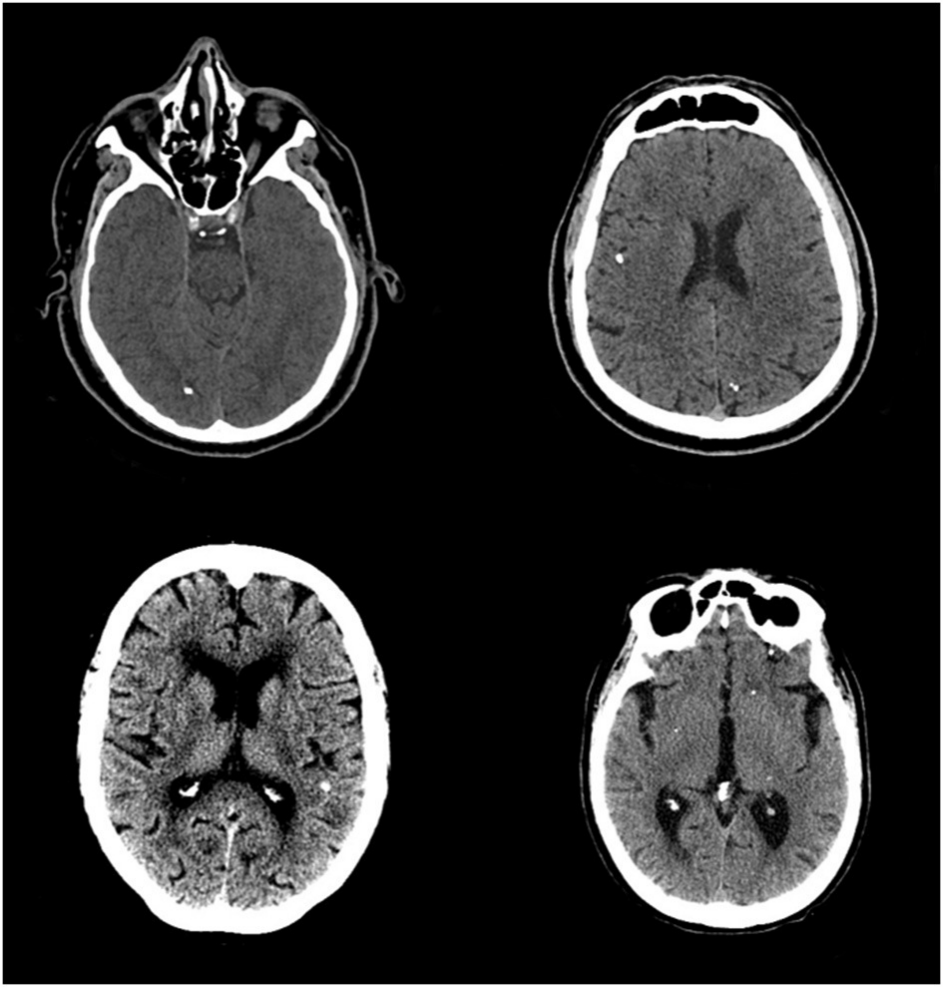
CT-scans of four different patients showing small calcifications (1–10 mm in diameter), single or multiple, located in brain parenchyma. These lesions are typically observed in patients with calcified neurocysticercosis and can be easily observed using CT-scan.
